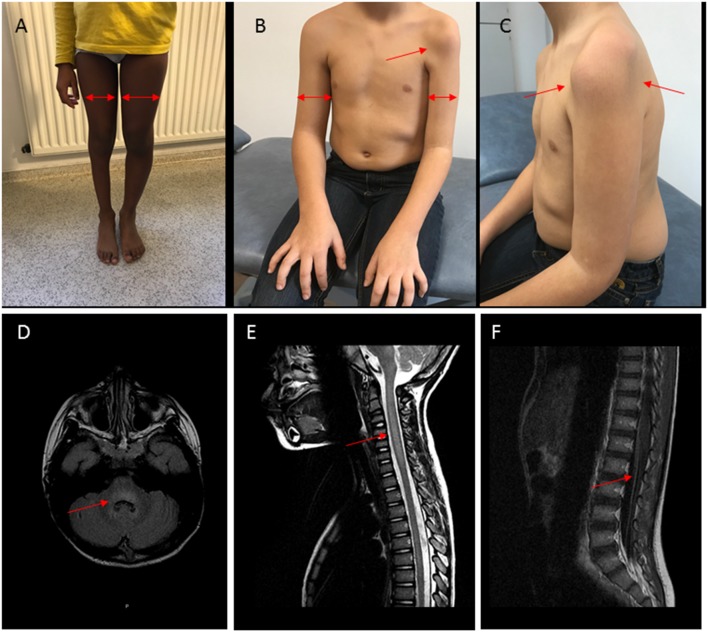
Clinical and MRI findings in patients with AFM and polio-like features. (A) Patient P3, right leg monoparesis, 3-years evolution. Proximal and distal muscular atrophy (arrows). (B,C) Patient P1, left arm monoparesis, 2-years evolution. Proximal and distal muscular atrophy (arrows). (D–F) Patient P3, MRI at the acute stage. (D) Hyperintensity (arrow) in T2FLAIR sequence with rhombencephalitis, (E) Hyperintensity and edema (arrow) in T2 sequence with myelitis, (F) vascular nerve roots enhancement (arrow).
